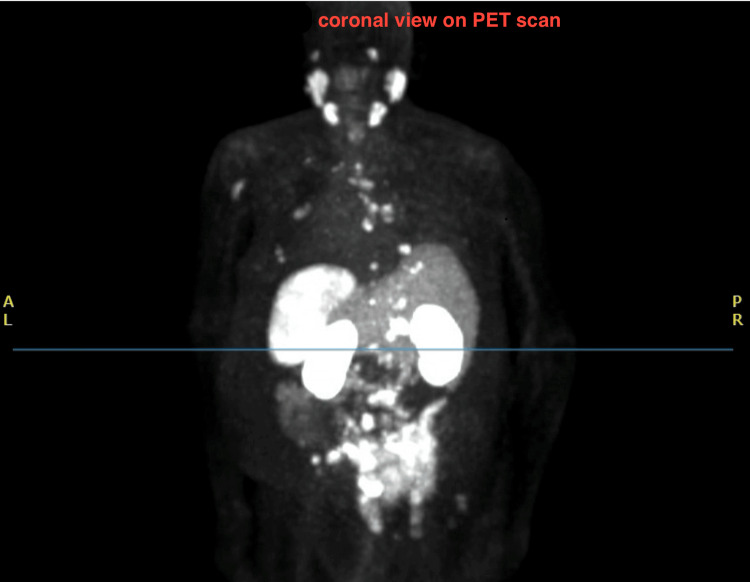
PET piflufolastat F-18 scan in coronal view showing uptake in multiple areas Piflufolastat F-18 PET scan is an FDA-approved PET scan against prostate-specific membrane antigen (PSMA)
